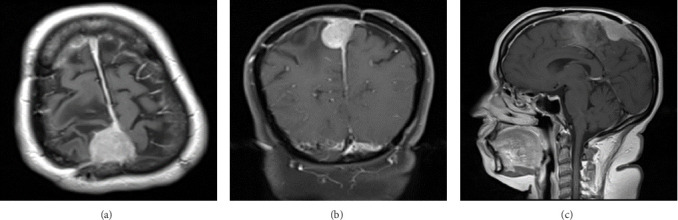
(A–C) Postoperative axial, coronal, and sagittal brain MRI following gadolinium administration. Tumor recurrence is noted at the previous site. It measures 2.8 cm × 2.6 cm × 2.5 cm in three dimensional planes. The tumor is infiltrating the adjacent superior sagittal sinus. MRI, magnetic resonance imaging.
